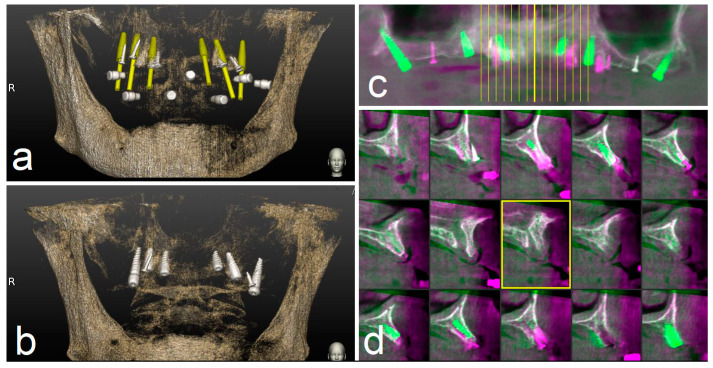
The preoperative surgical plan (a) and the postoperative cone beam computerized tomography (CBCT) (b) were superimposed using accuracy evaluation software (c,d). The registration was performed directly between the two volumetric images.
